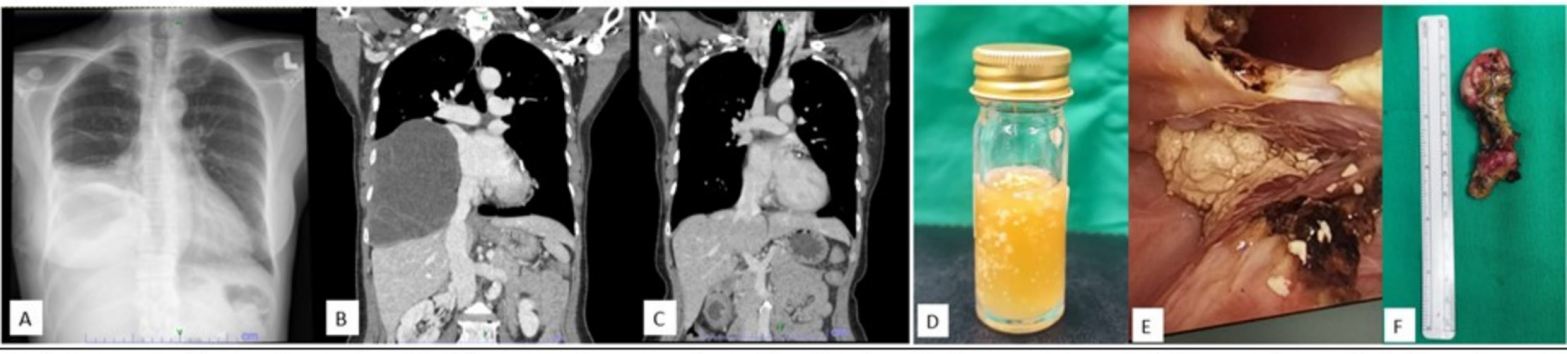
(A) Chest radiograph showed right moderate pleural effusion; (B) CECT coronal view showed right pleural effusion; (C) CECT surveillance post decortication showed resolving right pleural effusion; (D) Pleural fluid showed proteinaceous yellowish fluid; (E) Cheesy mass embedded in parietal pleural via video assisted thoracoscopy; (F) Pleural tissue with the tumour 8 cm length.
